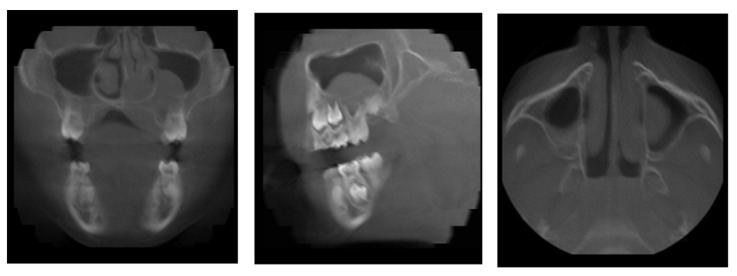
Patient, 8 y.o.: Coronal, sagittal, and axial reconstruction from CBCT examination. Soft-tissue mass covering the area of the hard palate and extending into a maxillary sinus and a nasal cavity.
